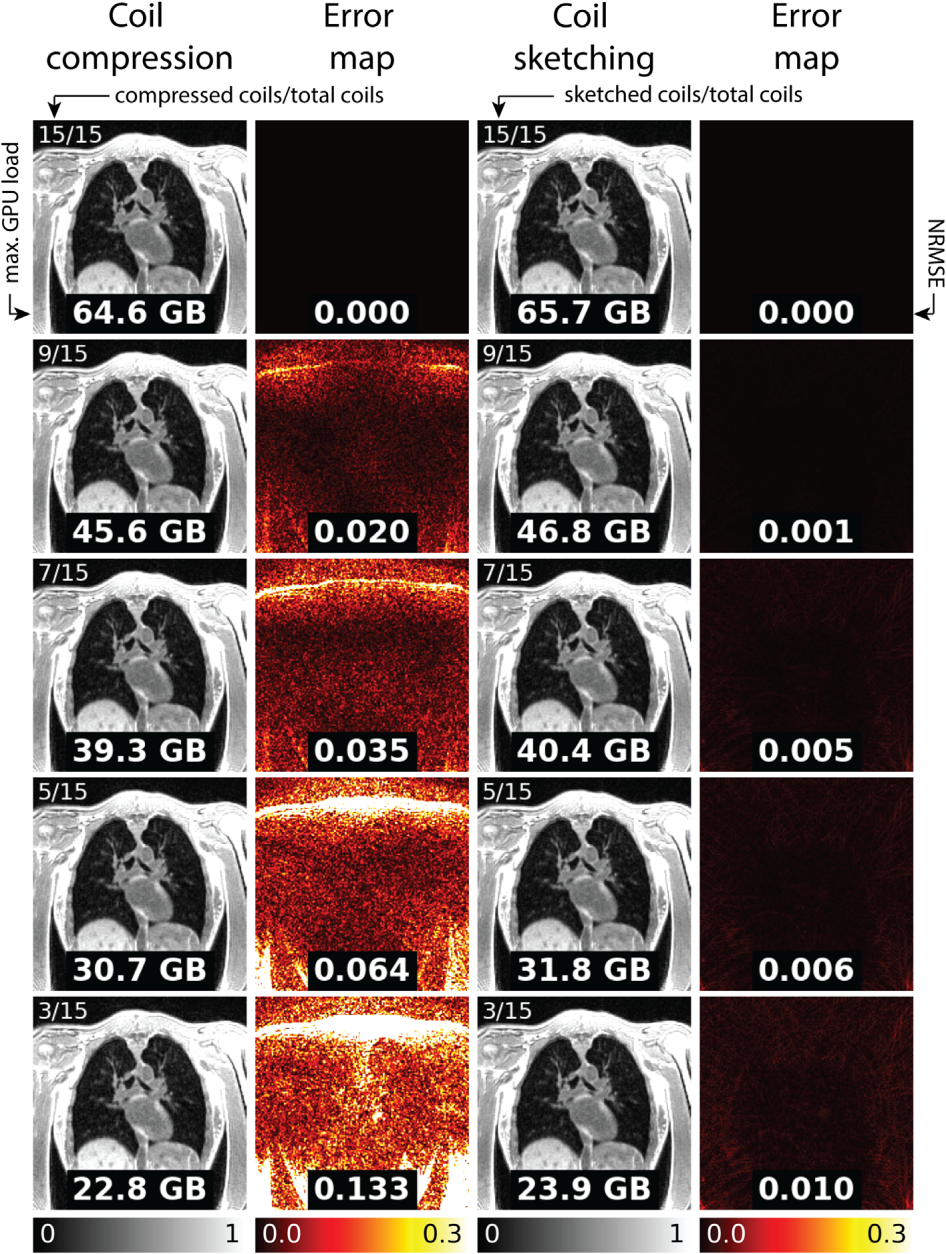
Comparison of memory footprint and accuracy between SVD coil compression and coil sketching reconstructions, with 3–15 coils, for 4D lung MRI (binned into 6 respiratory phases) using MoCo‐LR reconstruction. Each image has a maximum GPU memory allocation overlaid. Error maps are included with a normalized root mean square error (NRMSE) measurement overlaid.
